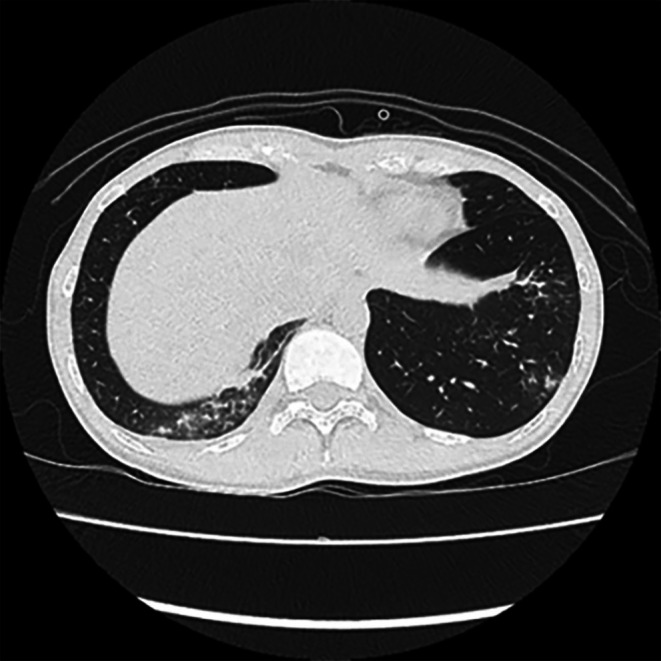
CT revealed consolidation and ground‐glass opacities in both lower lobes.
